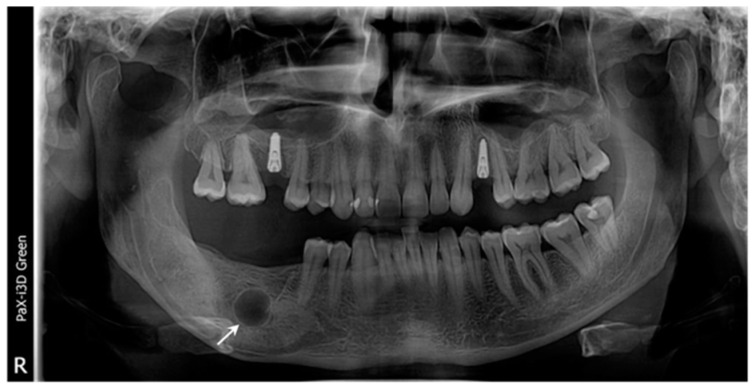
Orthopantomogram shows the second recurrence after one year, a unilocular radiolucency lesion, bounded by corticated well-defined margins (arrow), in the same place as the first operated recurrence.
